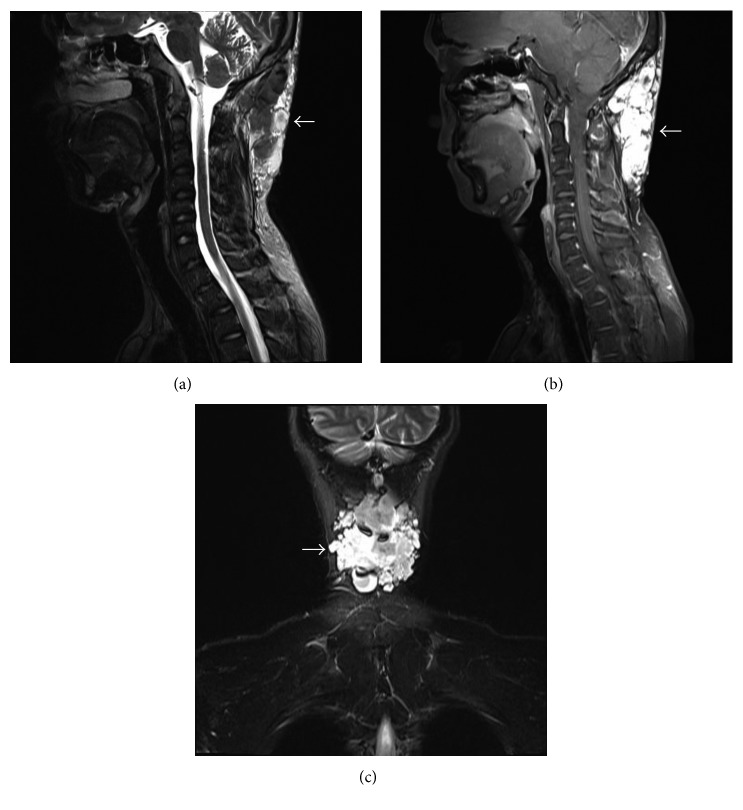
MRI demonstrated a mass (arrows) in the occipitocervical subcutaneous tissue with uneven signal intensity on (Figure 1(a)) sagittal T2-weighted, (Figure 1(b)) T2-weighted fat suppressed, and (Figure 1(c)) coronal enhanced images.
